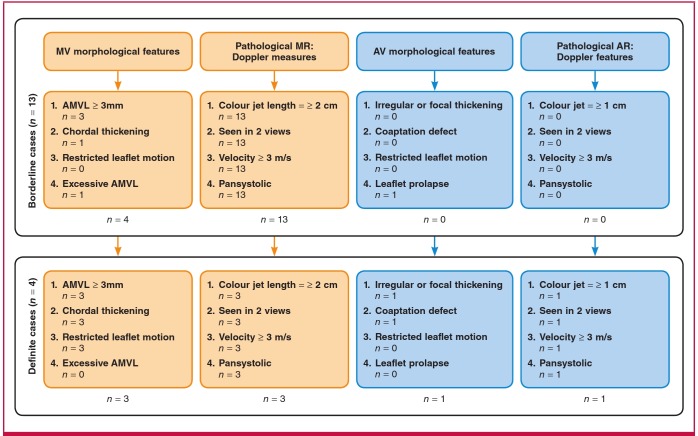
Children assessed as definite or borderline RHD using the morphological and Doppler criteria for RHD according to the WHF 2012 criteria.^9^
